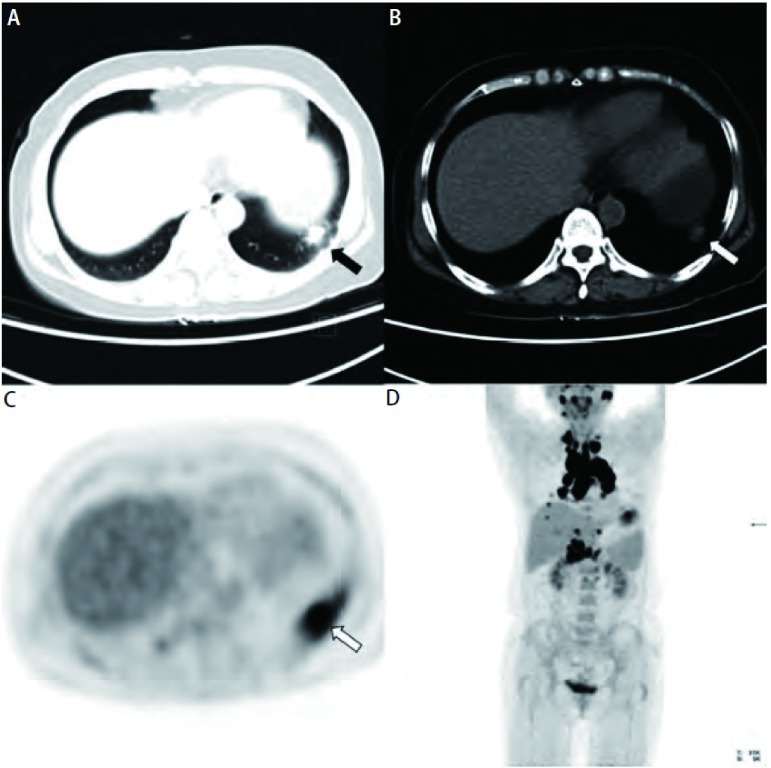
女，53岁，左肺下叶一枚结节性病灶，CT未见典型恶性征象（A、B），PET示异常浓聚（C），SUVmean: 8.07，按照肺内病灶初步诊断为可疑恶性，但全身PET/CT发现该病例双侧肺门肿大、纵隔、双侧颈部、腹膜后等部位的肿大淋巴结均呈FDG异常浓聚（D），根据其肺门淋巴结的“八字型”征象将诊断修正为结节病，最终经活检证实。 A female patient, 53 years old, CT demonstrates a nodule in his left lung but not seemed as malignancy (A, B, crude arrow). A hypermetabolic lesion with SUVmean value as 8.07 was identified on PET image (C, crude arrow). Preliminary diagnosed as probable malignancy. Whole body PET/CT showed FDG accumulate in bilateral hilar lymph nodes, mediastinal lymph nodes, bilateral cervical lymph nodes and retroperitoneal lymph nodes et al. (D). Modified diagnosis as sarcoidosis according to "八" sigh in hilar nodes, subsequently confirmed by biopsy.
